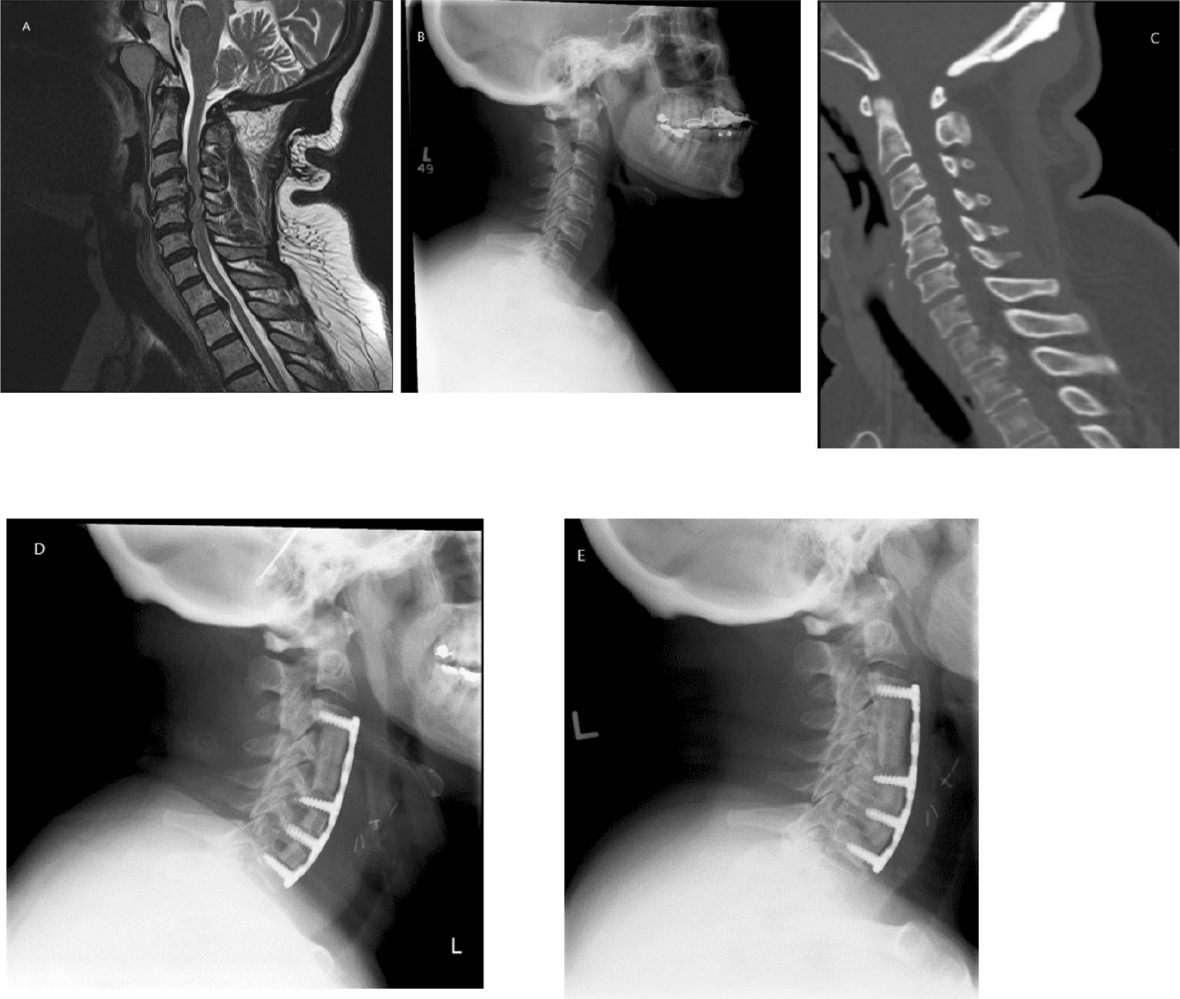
A 65-year-old female who presented with severe progressive cervical spondylotic myelopathy. MRI scan of the cervical spine showed multi-level cervical spondylosis with quite severe anterior cord compression, particularly at the C4/C5 level where there was a large partially sequestered disk (A). There was also significant spondylosis at C3/C4 and at C5/C6, and there was fairly significant degeneration of the foraminal narrowing at C6/C7 (B,C). The patient underwent a multilevel ACDF with C4 corpectomy (D). At the last follow-up 2 years postoperatively, X-rays of the cervical spine showed good alignment and solid fusion across each disc segment (E).
